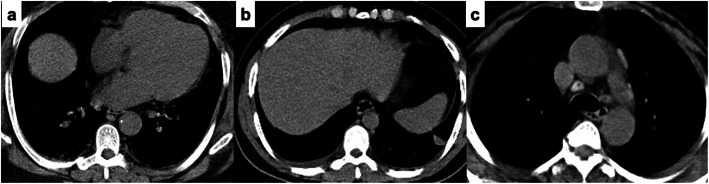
Axial CT mediastinal window in different cases with COVID-19 pneumonia. a Bilateral pleural thickening. b Right mild size free pleural effusion and left pleural reaction. d Mediastinal reactive lymph nodes noted at the right paratracheal, aortopulmonary, and prevascular regions. Note the high density of lymph nodes
